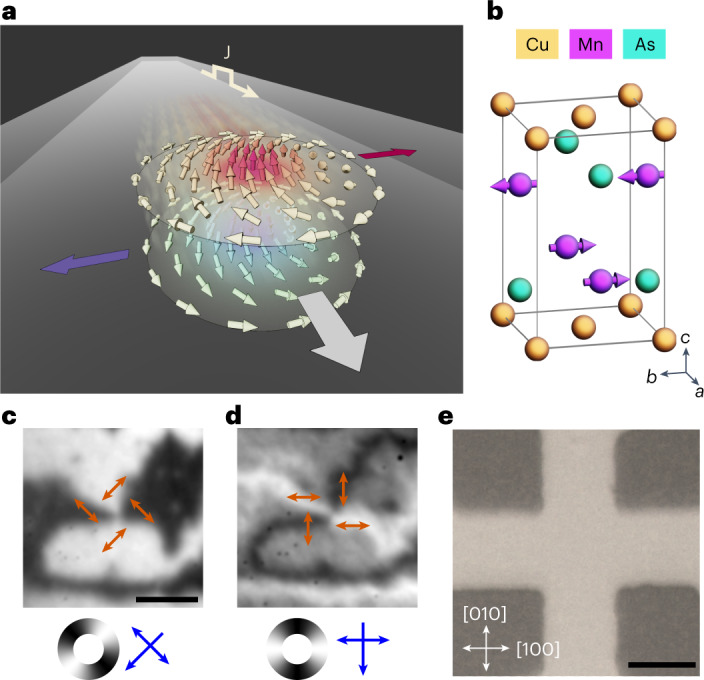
AF textures in CuMnAs. a, Spin structure and force acting on an AF Bloch-type meron under an applied current pulse J. b, Unit cell and magnetic structure of CuMnAs. c,d, XMLD–PEEM images of a vortex structure in CuMnAs. The blue single- and double-headed arrows indicate the X-ray incidence and polarization vectors, while the colour wheels and red double-headed arrows indicate the spin axis orientation inferred from the XMLD contrast. The scale bar corresponds to 1 μm. e, Optical image of the device structure used for electrical pulsing. The spatial scale bar corresponds to 10 μm.
